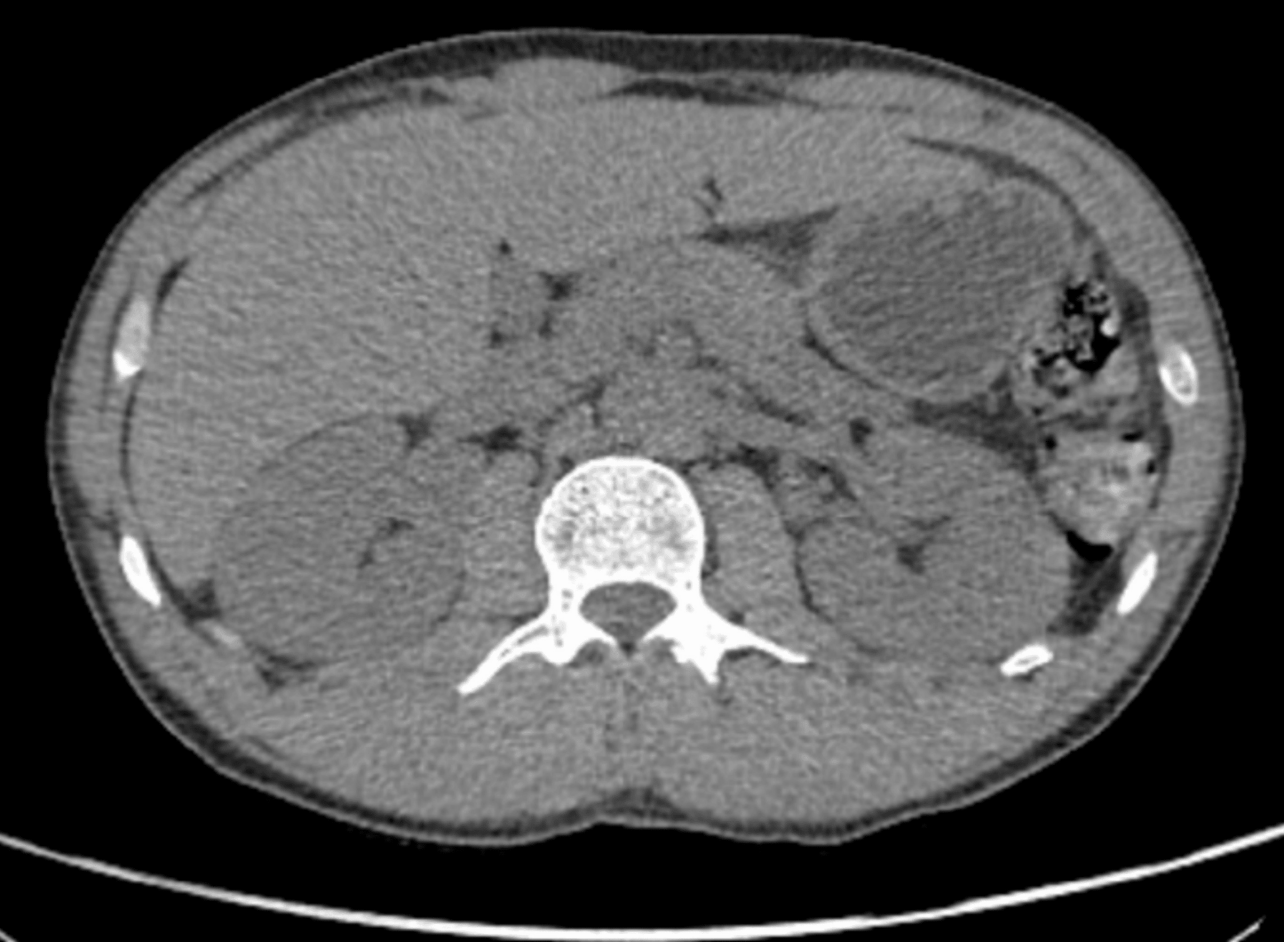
CT abdomen and pelvis (non-contrast) Findings: No hydronephrosis. No bowel obstruction or perforation or free fluid or collection. Bladder catheter is present. Diverticular disease of the large bowel. Normal non-enhanced appearance of the pancreas. No destructive bony lesion. Conclusion: Significant pathology may not be demonstrated on these images. There is no bowel obstruction or perforation and no hydronephrosis.
